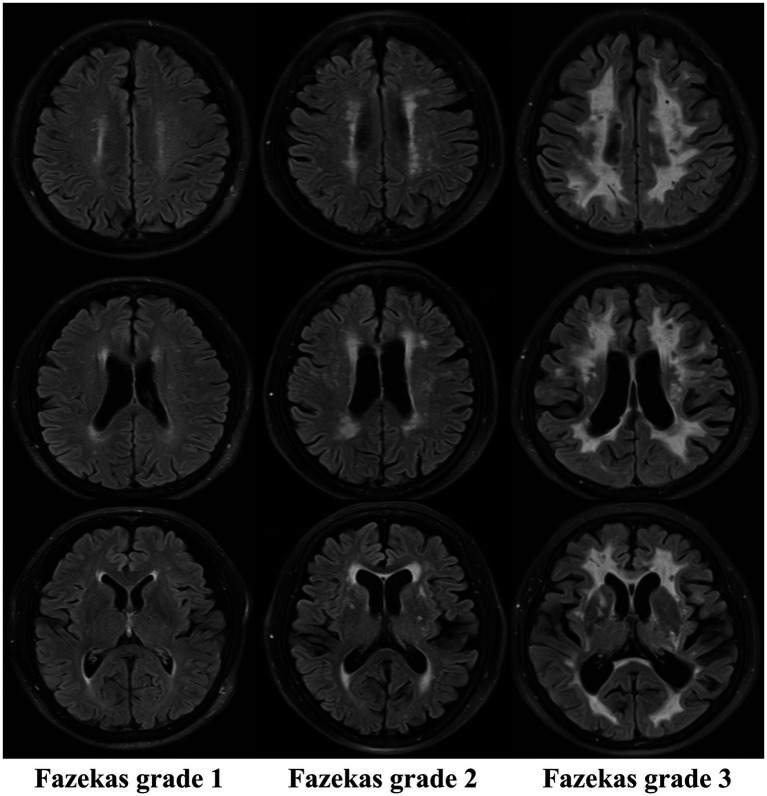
Characteristic MR imaging findings on white matter hyperintensity lesions (Fazekas).
